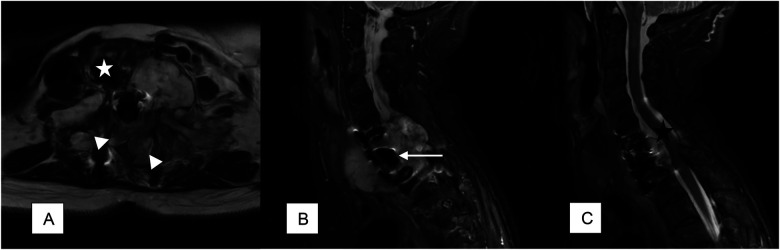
MRI shows massive tumor growth 11 years after the first intervention. (A) The lesion extends to the whole C7 residual vertebra, also involving the adjacent C6 and T1, and is characterized by a bilateral high-grade invasion of the spinal canal (white arrowheads) and an anterior exophytic growth on the left side dislocating the trachea (white star) controlaterally; (B,C) the tumor entirely envelopes the previously implanted expandable cage (white arrow) and compressed and dislocated the spinal cord (black star).
